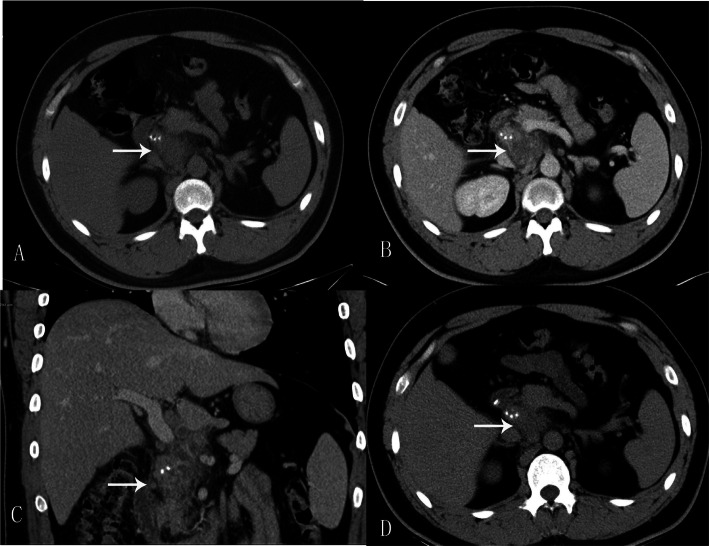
a Computed tomography scan without contrast showing irregular soft tissue mass (*White arrow*). The lesion had an uneven interior and was surrounded by a blurred fat gap. There were multiple spots of high density with clear margins in the upper right of the lesion. b-c Contrast-enhanced computed tomography showing multilocular changes of the lesion (*White arrow*), uneven enhancement of cystic wall, slight enhancement of adjacent duodenal wall, and multiple enlarged lymph nodes. d Abdominal computed tomography was reviewed 1 month after treatment. Computed tomography scan without contrast showing the postoperative change of local high-density imaging in the duodenum wall, the volume of the lesion (*White arrow*) gradually decreased, and the edge was clear, and no substantial changes in the multiple high-density shadow spots were seen
